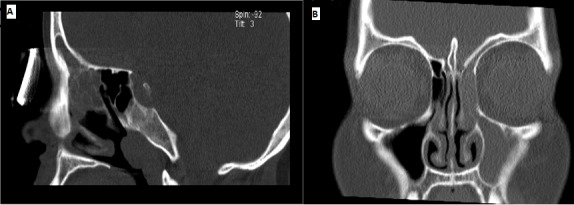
A) sinonasal CT coronal and B) sagittal views in a patient with SNS shows mucosal thickenings of the left maxillary, anterior ethmoidal, and the frontal sinuses as indicated by arrows.
